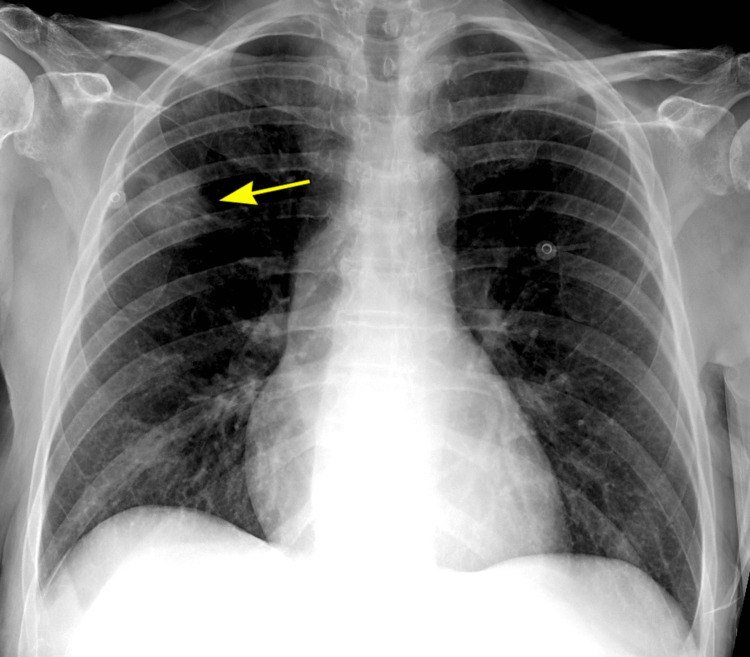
Chest X-ray showing the patient’s right upper lobe mass concerning for malignancy (yellow arrow)
